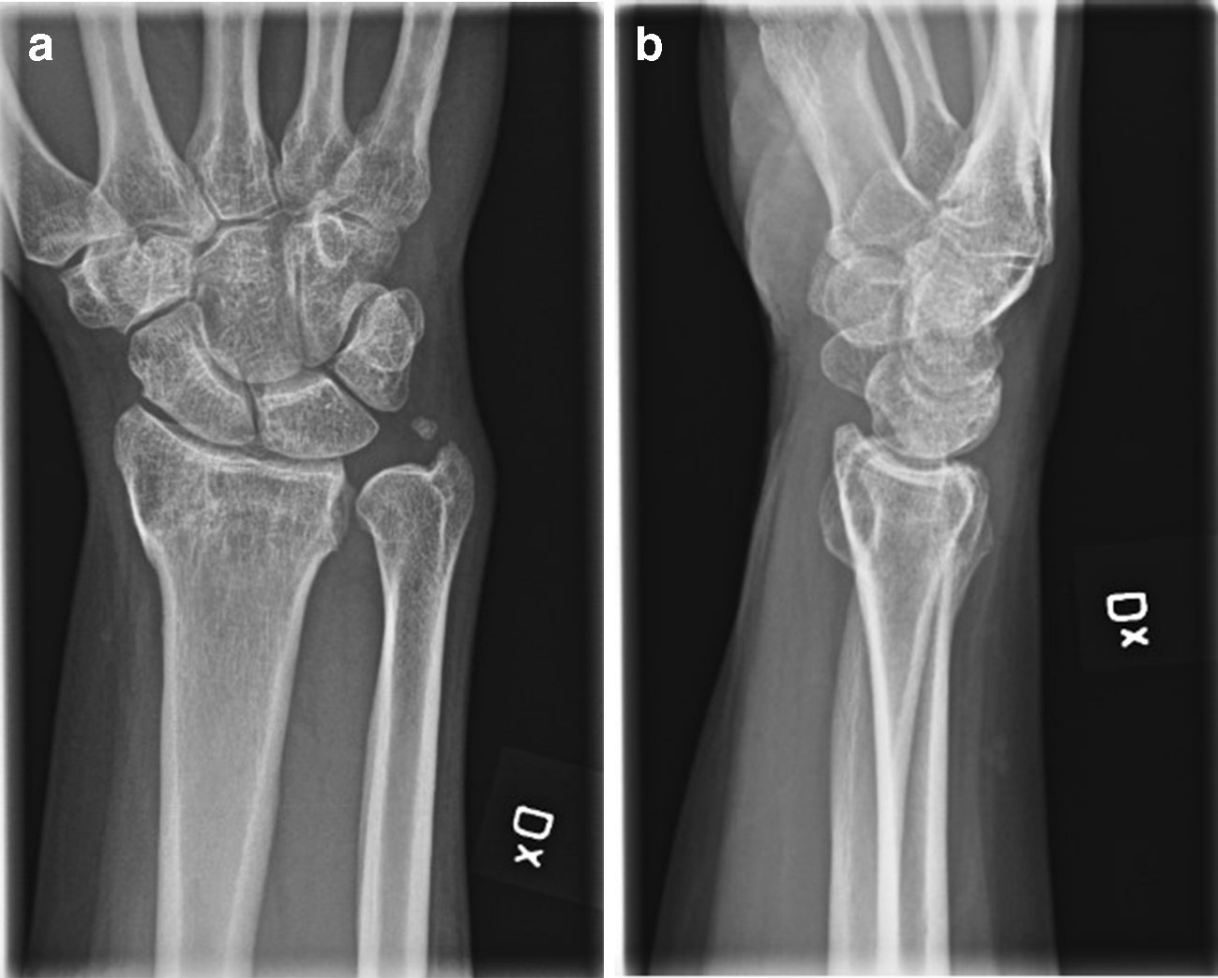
Pre-operative radiograph of malunited distal radial fracture scheduled for osteotomy with bone substitute. a AP view. b Sagittal view
